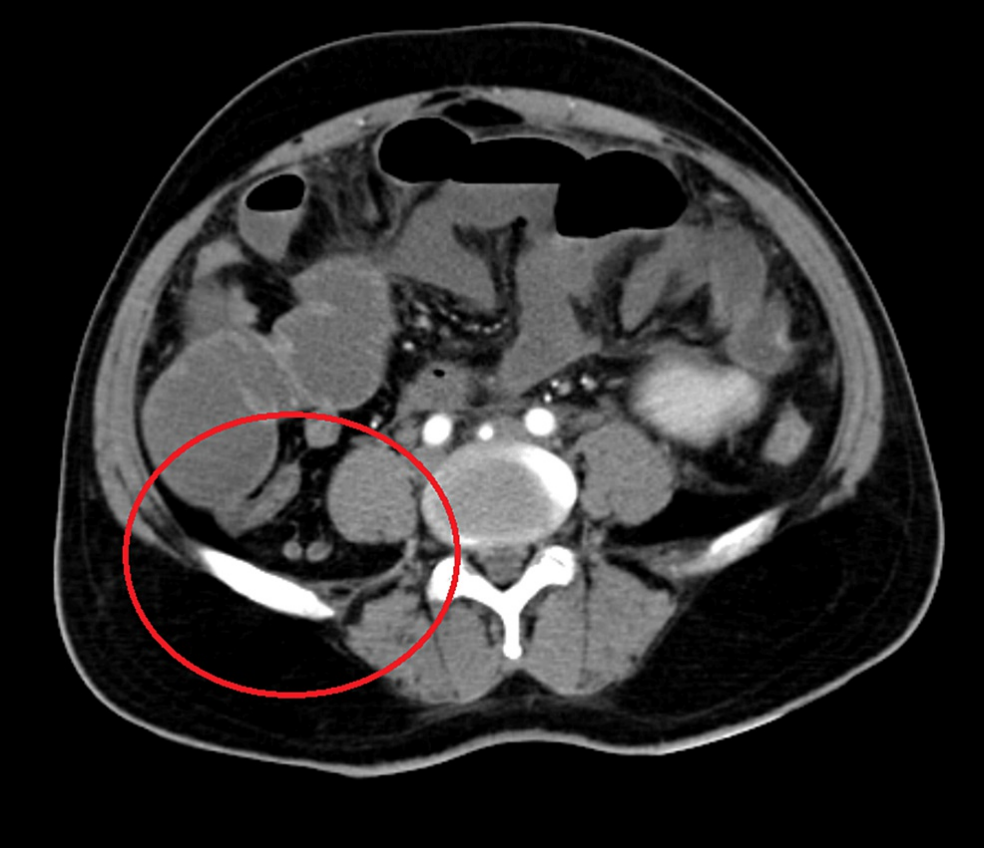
CECT abdomen-red encircled part showing transition point of intestinal obstruction in the distal ileal loop CECT: contrast-enhanced computed tomography
